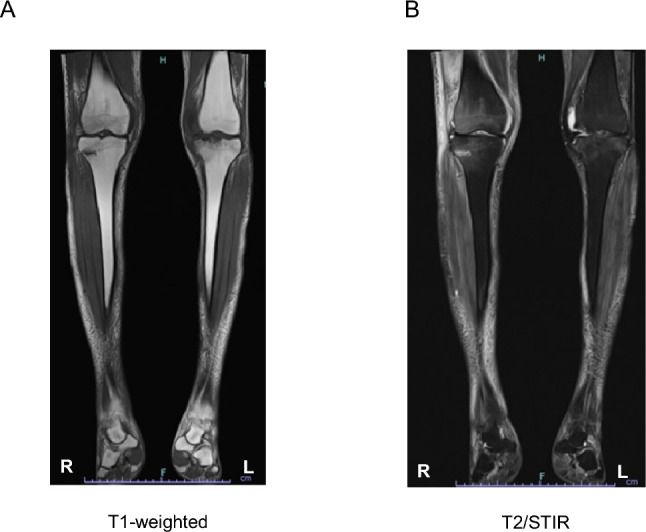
T1-weighted (A) and T2/STIR (B) MRI images of the right and left knees
